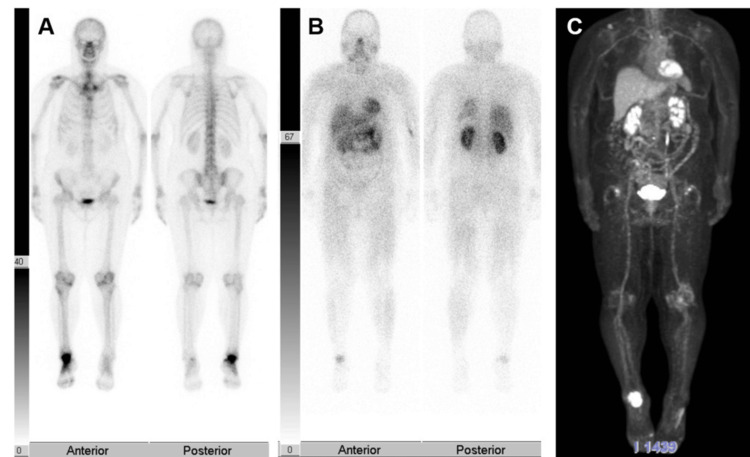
Bone scintigraphy, Thallium scintigraphy, Positron Emission Tomography Computed Tomography (PET-CT)
